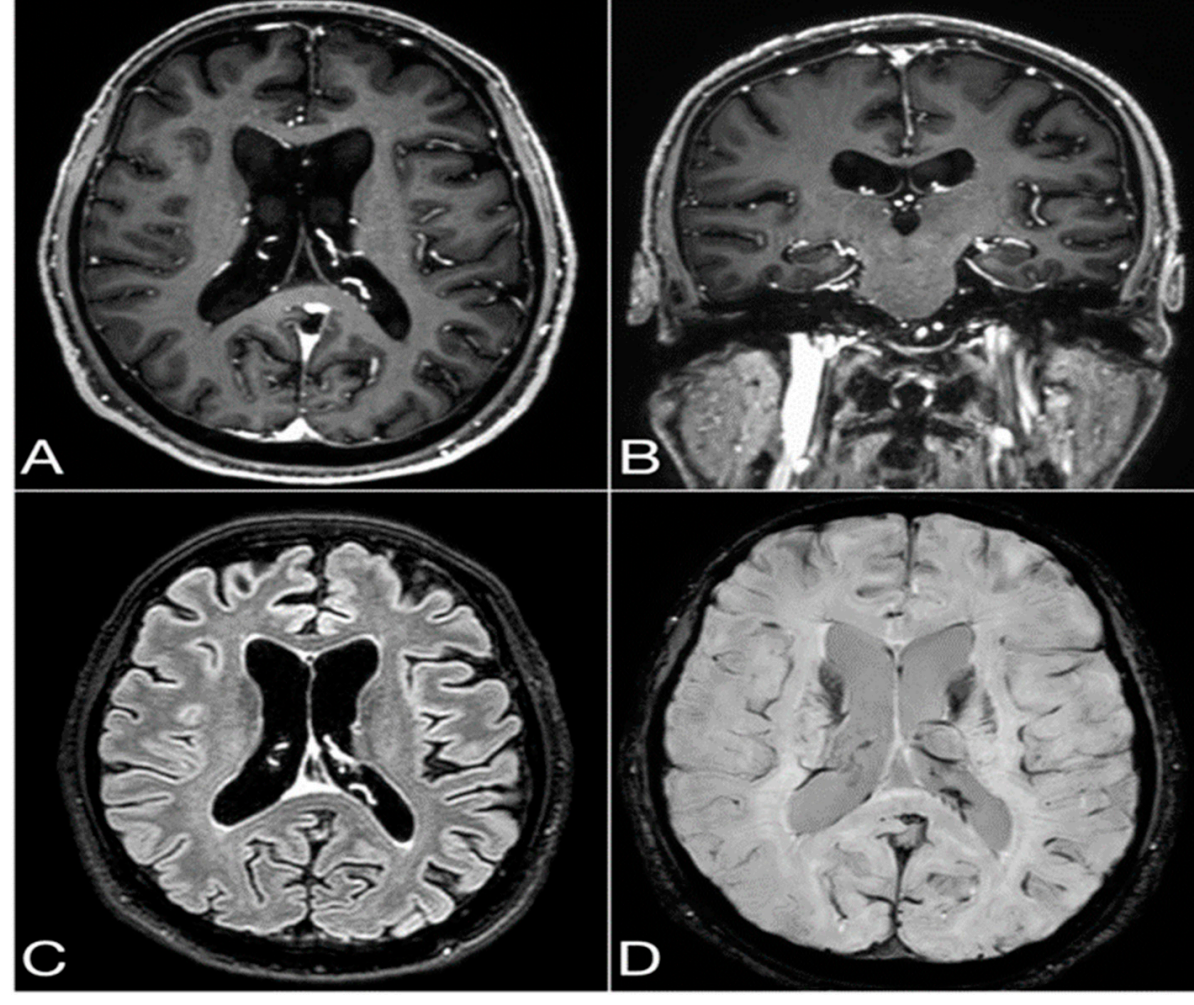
Last brain MRI performed with a 3T scanner (Philips Ingenia) with a 32-channel phased-array head coil in March 2024, showing moderate to severe signs of cortical atrophy and ventricular enlargement, with no sign of cerebral vasculopathy (neither leukoaraiosis nor microbleeds) or pathological post-contrast enhancements. Axial (A) and coronal (B) 1 mm thick multiplanar reconstructions from 3D T1-weighted Turbo Field Echo (TFE) with fat saturation and axial (C) 1 mm thick multiplanar reconstruction from 3D T2-weighted Fluid-Attenuated Inversion Recovery (FLAIR) with fat saturation (both sequences acquired after i.v. injection of gadolinium-based MRI contrast agent). Axial susceptibility-weighted imaging (SWI) processed magnitude image with a thickness of 2 mm (D).
